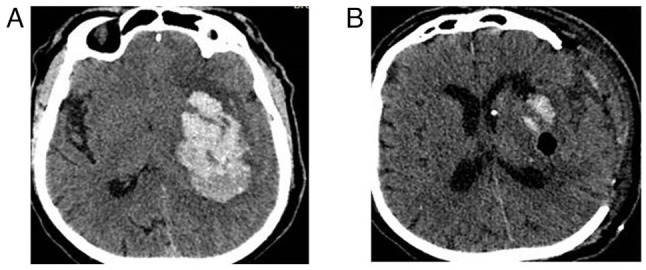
Fronto-temporoparietal craniectomy in a patient with intracerebral hemorrhage (female patient, 52 years of age, with intracerebral hemorrhage into the left side underwent left-side craniectomy and right hemiplegia). (A) Pre-operative CT scan, axial view; (B) post-operative CT scan, axial view.
